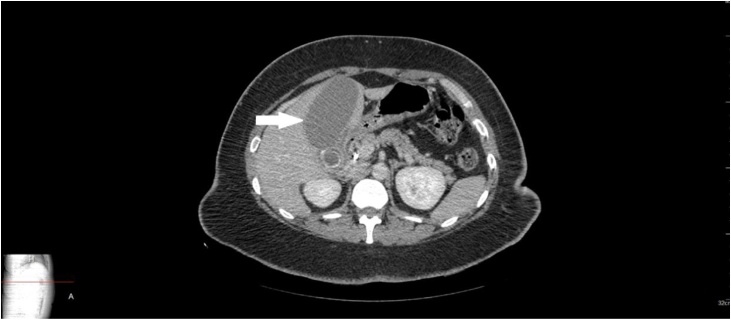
CT scan showing distended gallbladder with an impacted stone in neck/cystic duct.
